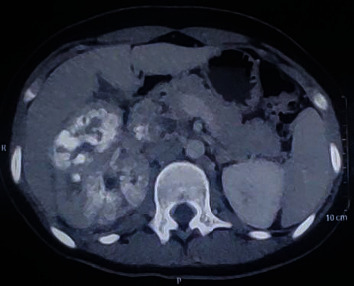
Axial CT scan showing right renal calcifications with involvement of IVC.
